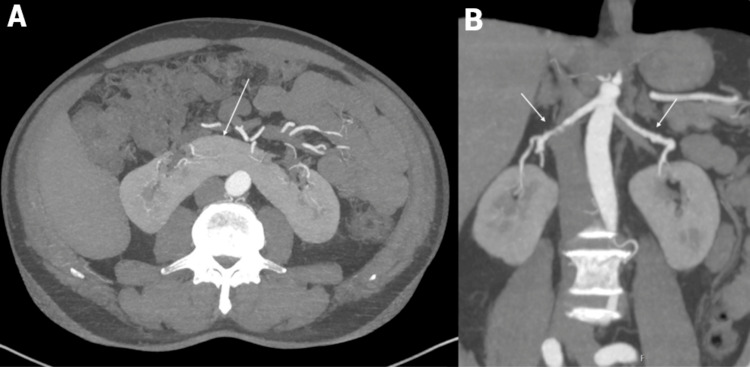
CT scan Contrast-enhanced CTA of the abdomen. (A) Axial reconstruction revealing a horseshoe kidney with fusion of the lower poles of both kidneys in the midline (arrow). (B) Coronal reconstruction with a typical string-of-beads appearance of both renal arteries (arrows), which is characteristic of multifocal FMD. CTA, CT angiography; FMD, fibromuscular dysplasia
